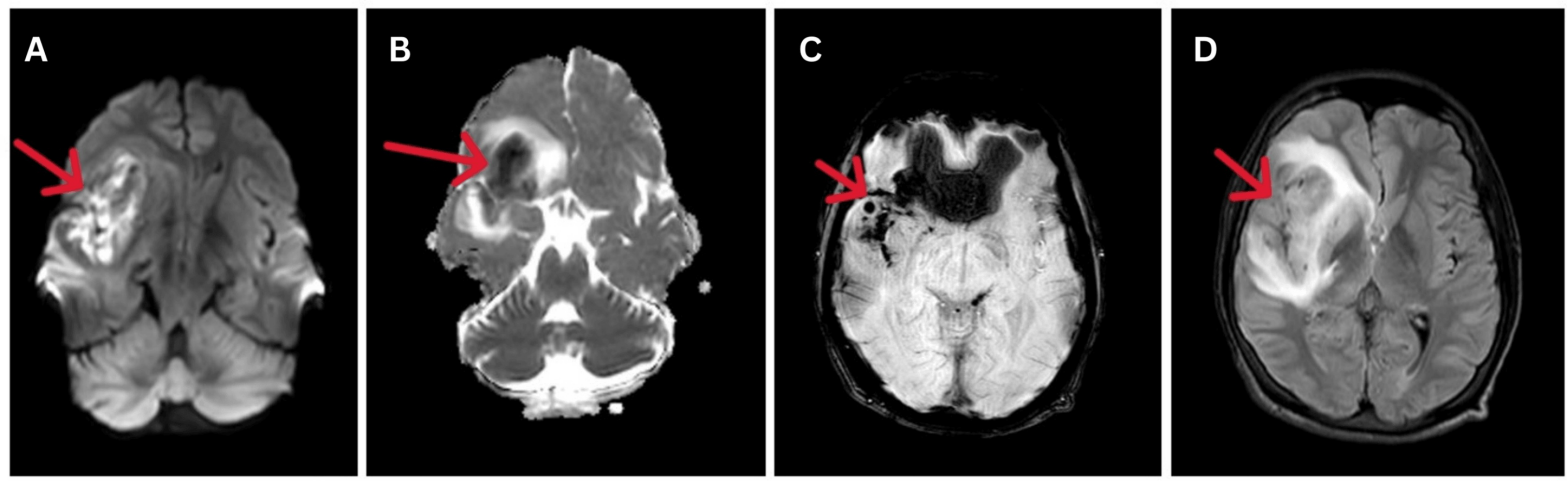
(A) Diffusion, (B) ADC, (C) SWI, and (D) FLAIR sequences of MRI of the brain suggestive of acute infarct with haemorrhagic transformation in the right temporal-frontal lobes (red arrows). ADC: apparent diffusion coefficient; SWI: susceptibility-weighted imaging; FLAIR: fluid-attenuated inversion recovery; MRI: magnetic resonance imaging
